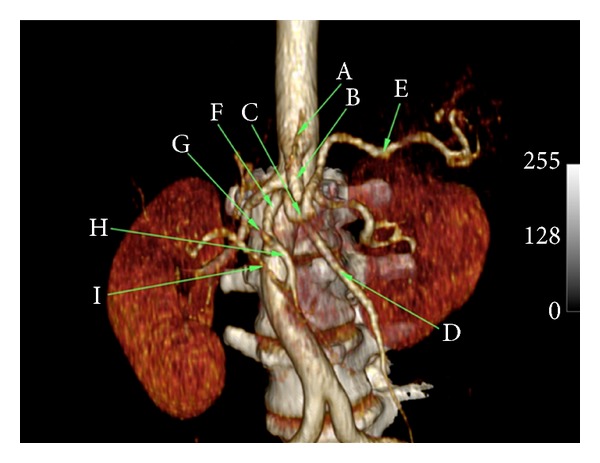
Reconstructed coronal CT images demonstrating the new anatomic variant. Key: left gastric artery—A; celiacomesenteric trunk—B; coeliac trunk—C; superior mesenteric artery—D; splenic artery—E; common hepatic artery—F; left hepatic artery—G; gastroduodenal artery—H; right hepatic artery—I.
